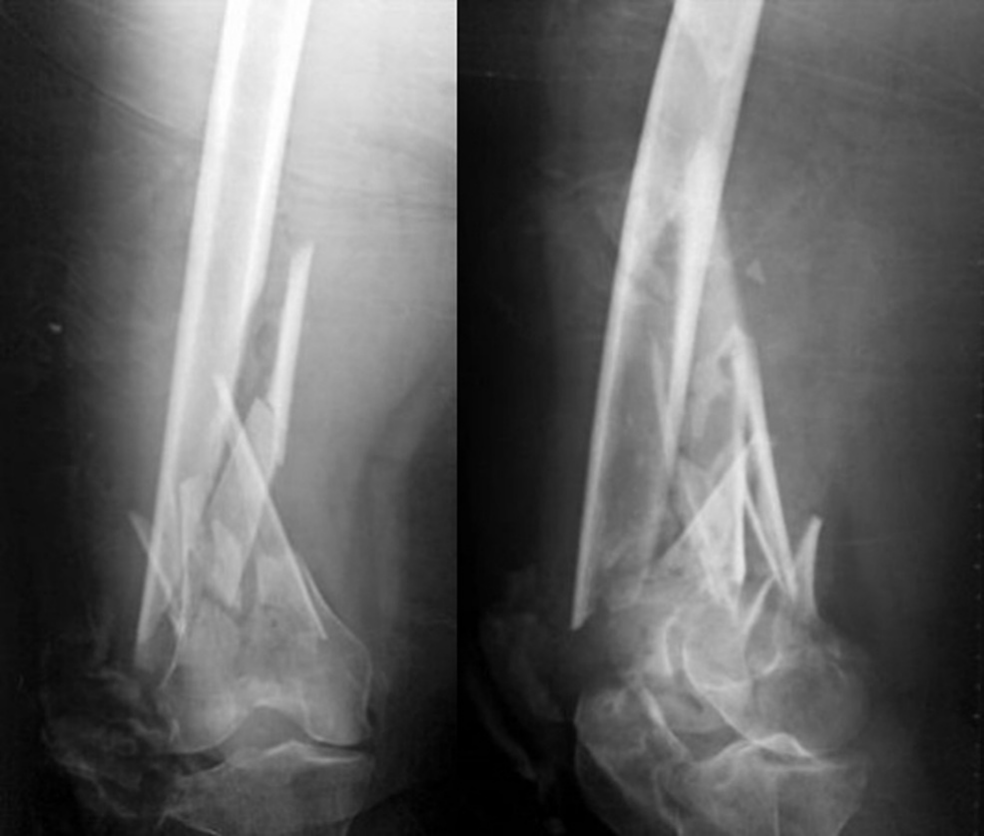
Pre-operative x-rays. X-rays show a comminuted (AO type C2) supra-condylar femur fracture with intra-articular extension.
